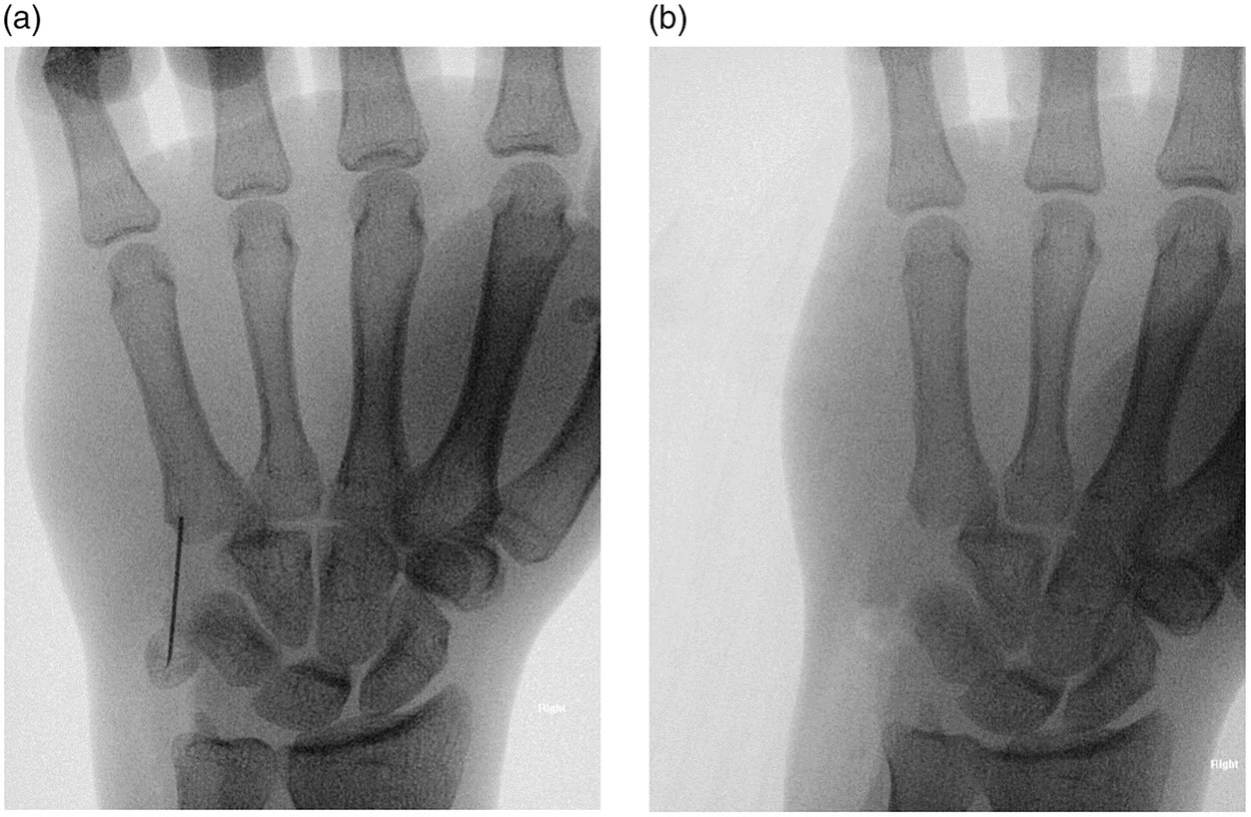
(a) X-ray (anteroposterior view) of the right hand before complete excision and removal of the comminuted pisiform. (b) X-ray (lateral view) of the right hand after complete excision and removal of the comminuted pisiform.
